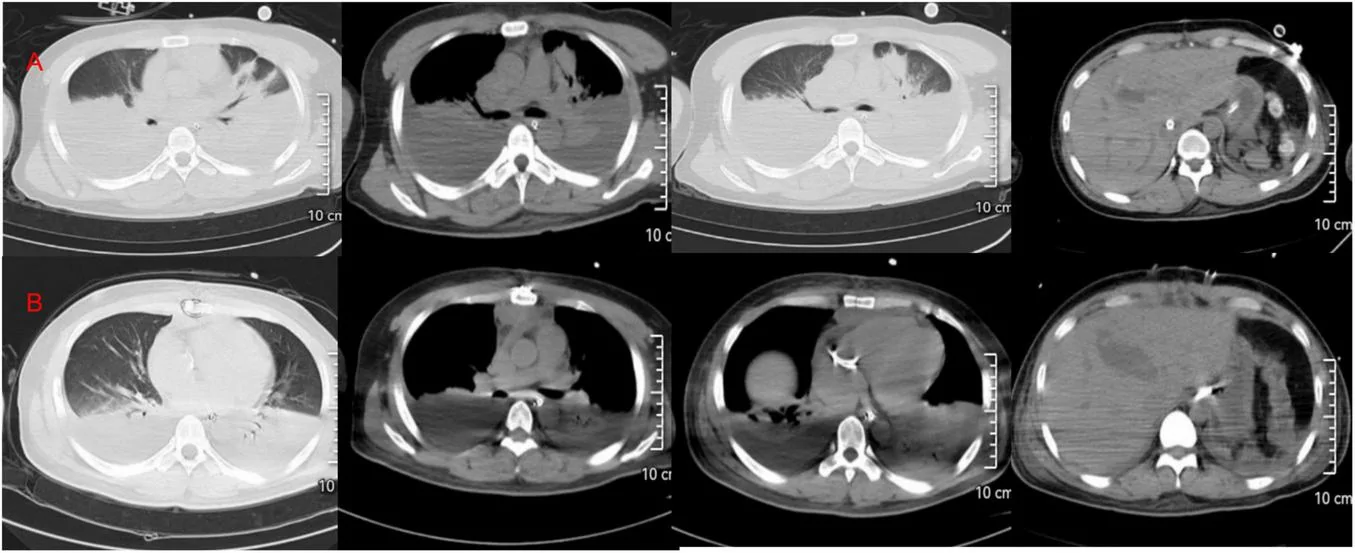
Computed tomography (CT) images of the chest. (A) Chest CT performed on the day of transfer to our hospital revealed bilateral patchy high-density shadows and partial bronchial involvement, accompanied by massive bilateral pleural effusions. A small amount of ascites was noted. (B) Chest CT performed on postoperative day 3 demonstrated improvement in pulmonary infection and atelectasis, as well as a decrease in bilateral pleural effusions.
